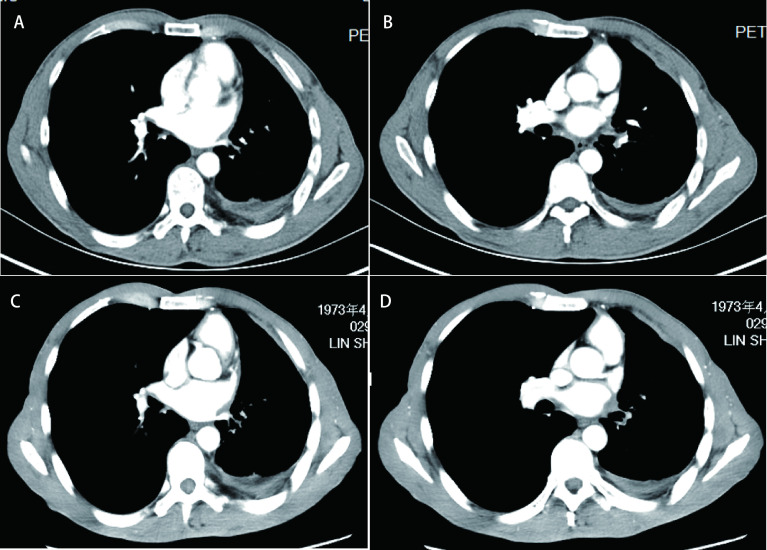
恩沙替尼治疗期间CT图像。A：CT(2019年11月13日)：左侧少量胸腔积液; B：CT(2019年11月13日)：纵隔淋巴结，左肺下叶小病灶; C：CT(2020年08月20日)：左侧胸腔积液同前; D：CT(2020年08月20日)：纵隔淋巴结同前，左肺下叶病灶同前。 CT images during treatment with ensartinib. A: CT (13-Nov-2019): a small pleural effusion on the left; B: CT (13-Nov-2019): mediastinal lymph node, small lesion in the lower lobe of the left lung; C: CT (20-Aug-2020): the small pleural effusion on the left was as same as before; D: CT (20-Aug-2020): the mediastinal lymph node was as same as before, the small lesion in the lower lobe of the left lung was as same as before.
